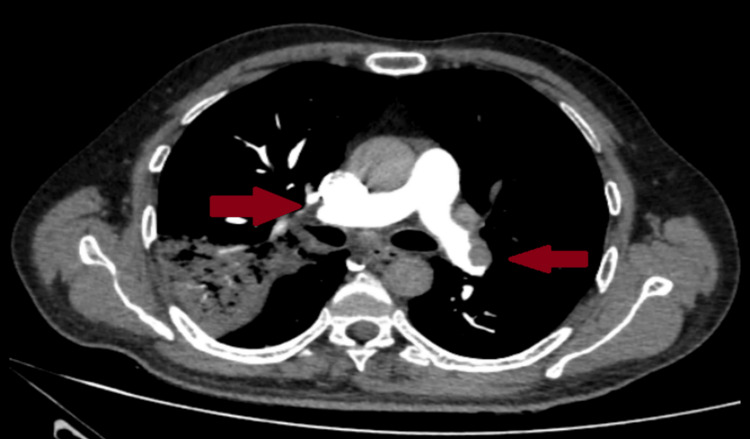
Computed tomography pulmonary angiography showing a sub-massive thrombus in major arteries as depicted by red arrows
